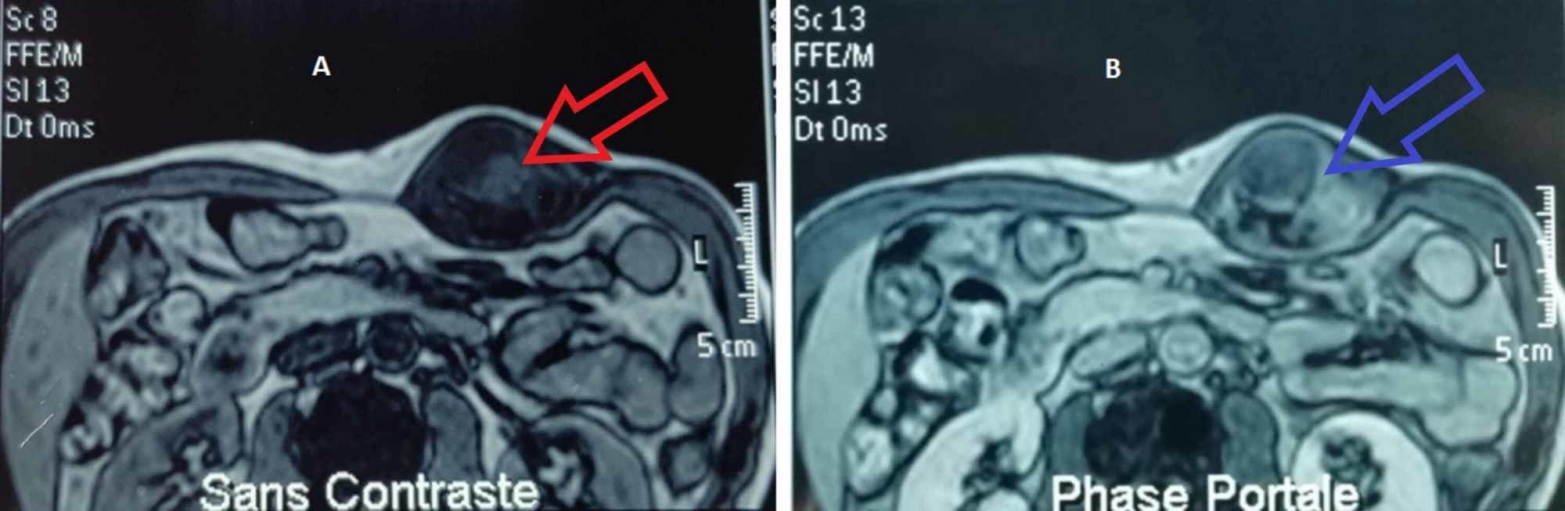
(A) Abdominal MRI showing a hypointense tumor (red arrow) on T1-weighted image. (B) The gadolinium contrast enhancement image shows a predominantly adipose mass containing nodular non-lipomatous components (blue arrow).
